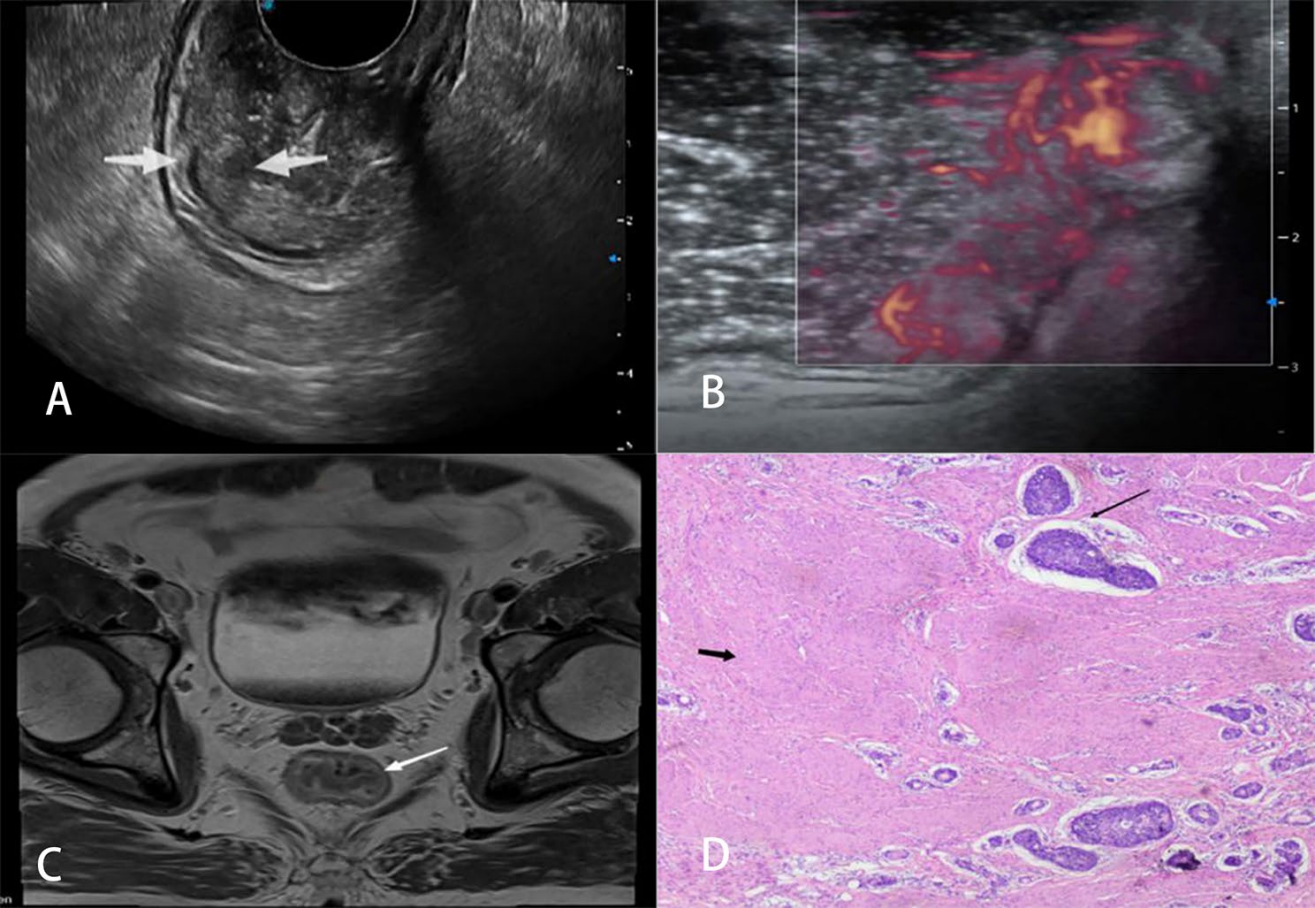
Images of a 70-year-old man with a T2 stage rectal tumor. (A) Two-dimensional endorectal biplane ultrasound of the convex array revealed a hypoechoic mass on the rectal wall that penetrated the submucosa and was localized in the muscularis propria (arrows); the diagnosis was T2 rectal cancer. (B) An MFI mode image revealed that the microblood flow signals of the mass were abundant; there were more than four blood flow streams that spread to more than 50% of the maximum section of the tumor. (C) Magnetic resonance imaging revealed thickening of the wall of the lower rectum under peritoneal reflection (arrow); this was diagnosed as T2 rectal cancer. (D) The pathological diagnosis was moderately differentiated adenocarcinoma stage T2 as ulcerative type; the cancerous tissue (long arrow) had invaded the superficial muscularis propria (short arrow) and was confined to the muscularis propria (the entire view is filled with the muscularis propria)
